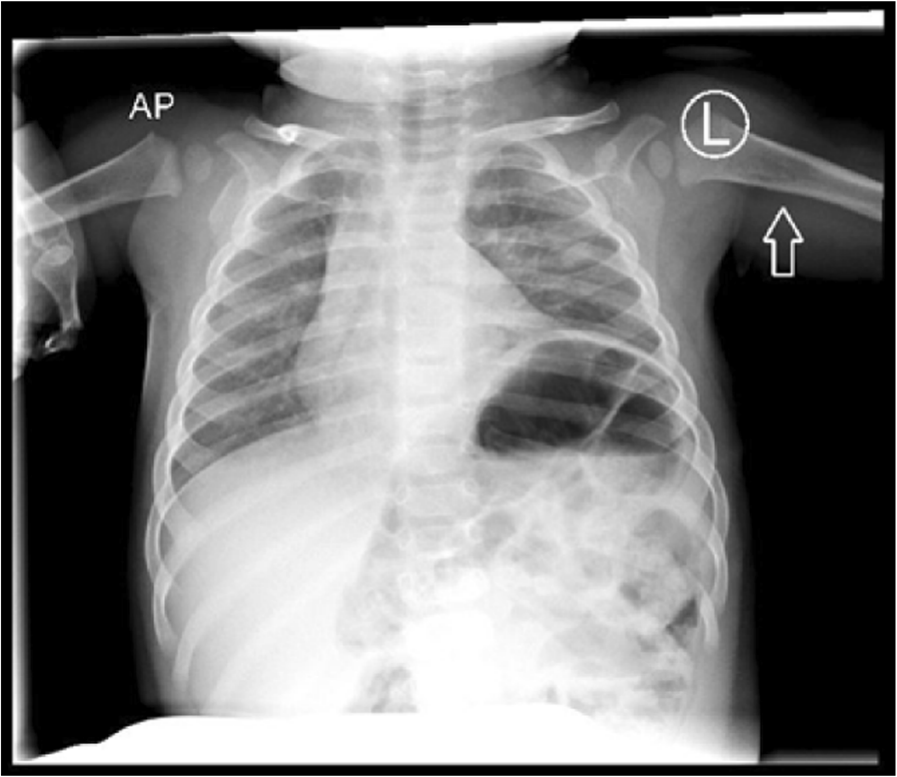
Post-operative Chest X-Ray. Persistent asymptomatic left hemidiaphragm elevation demonstrated on post-operative chest X-ray
